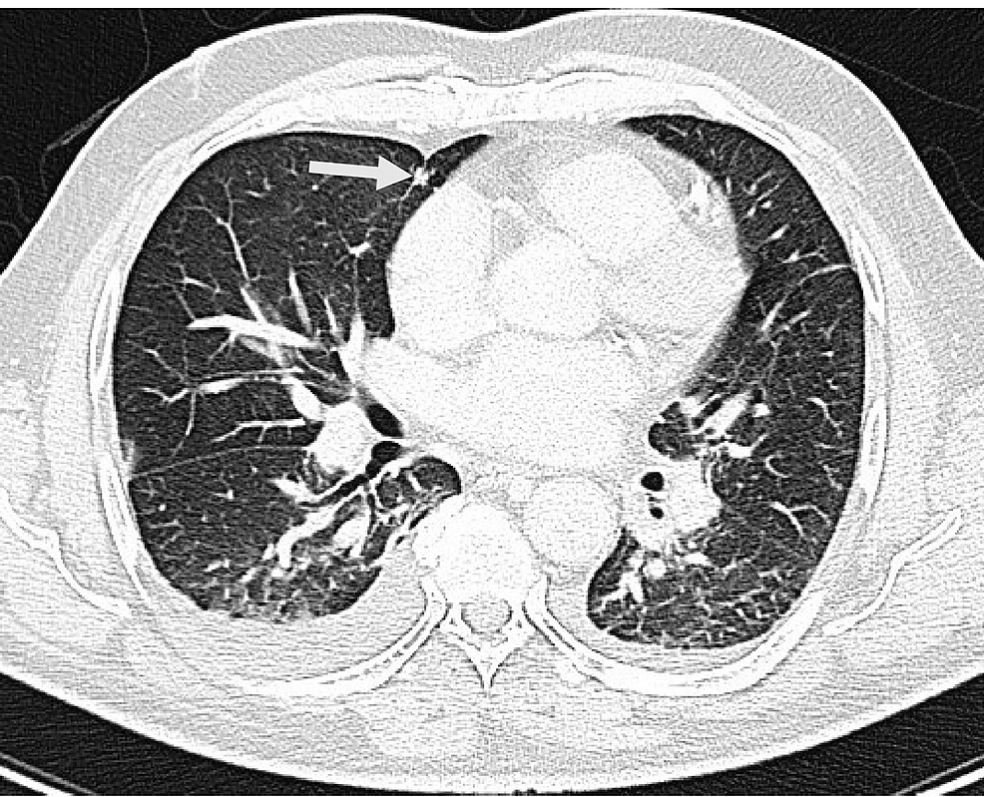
CT scan chest of the same patient’s lung window showing a small nodule (arrow) 5 mm at the middle lobe on the right side.
